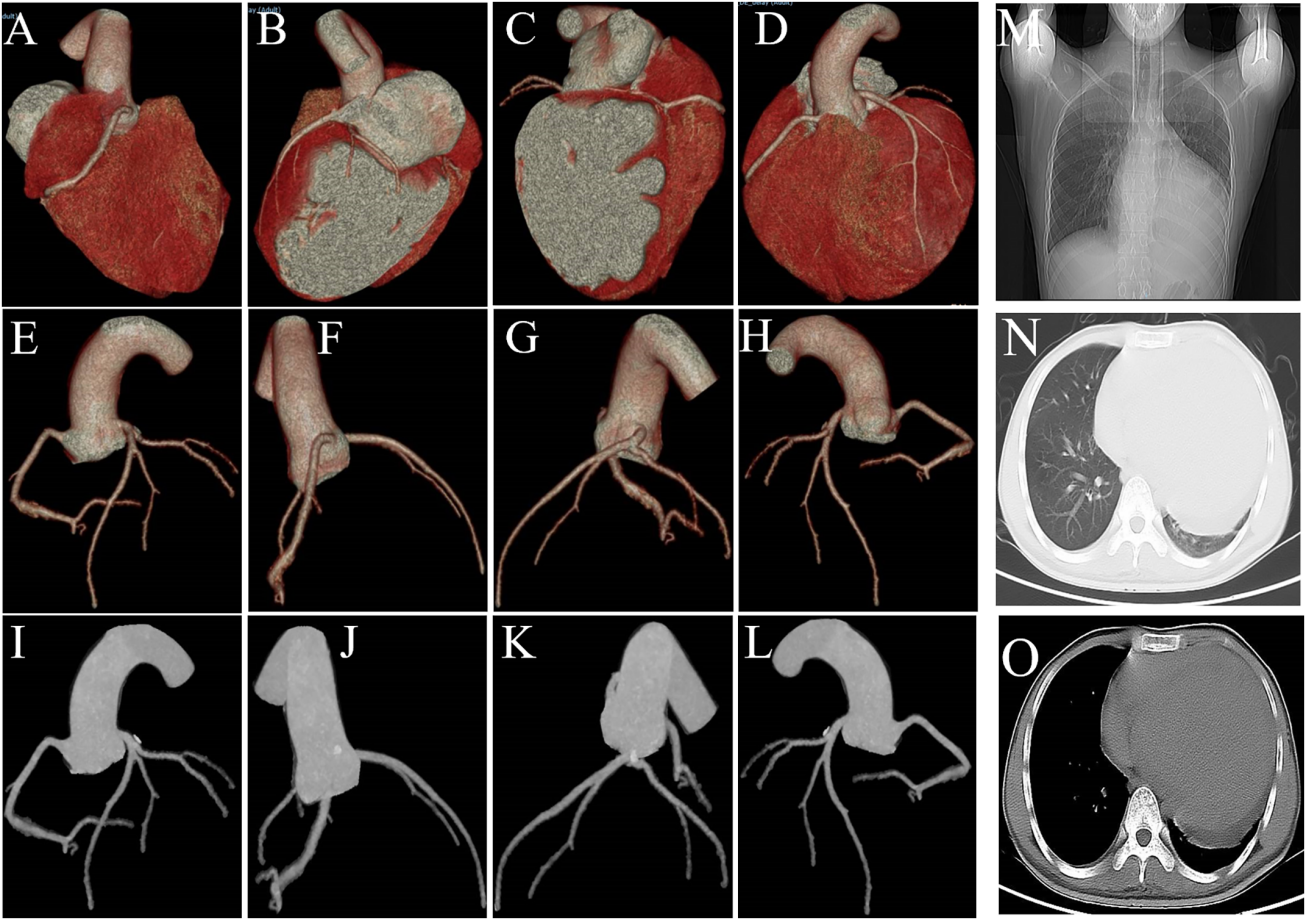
(A–L) CT coronary angiography shows no significant stenosis or filling defects. (M–O) The CT pulmonary scan reveals inflammation in the lower lobe of the left lung and cardiomegaly.
